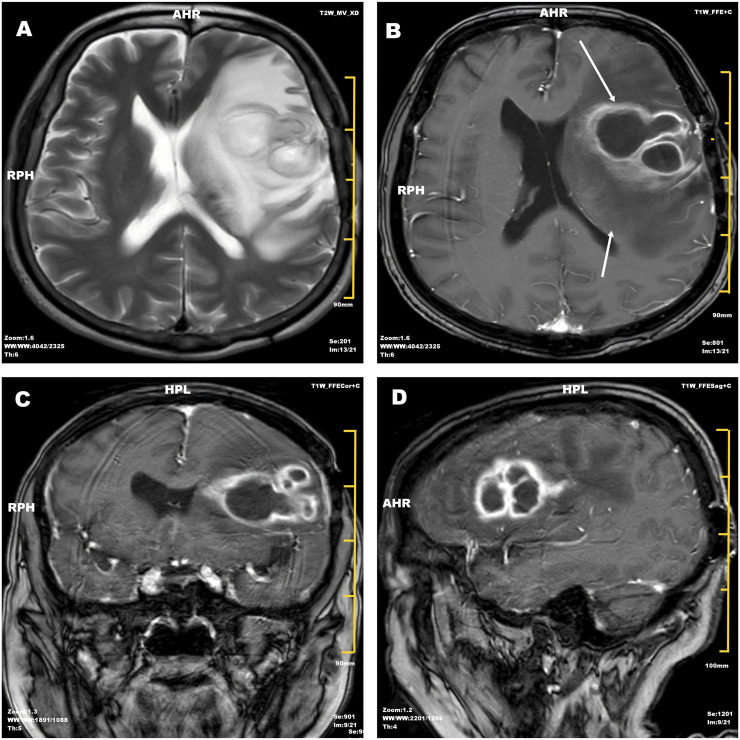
Cranial magnetic resonance imaging demonstrating brain abscess. (A) Diffusion-weighted imaging showing a 47 × 31 × 43 mm hyperintense lesion in the left frontal lobe with restricted diffusion. (B) T1-weighted post-contrast image revealing peripheral ring enhancement (arrowheads) with surrounding edema. (C) Apparent diffusion coefficient map demonstrating hypointense signal, confirming restricted diffusion. (D) Coronal FLAIR sequence showing mass effect with 6 mm midline shift. MRI: 3.0 T; gadolinium 0.1 mmol/kg IV.
